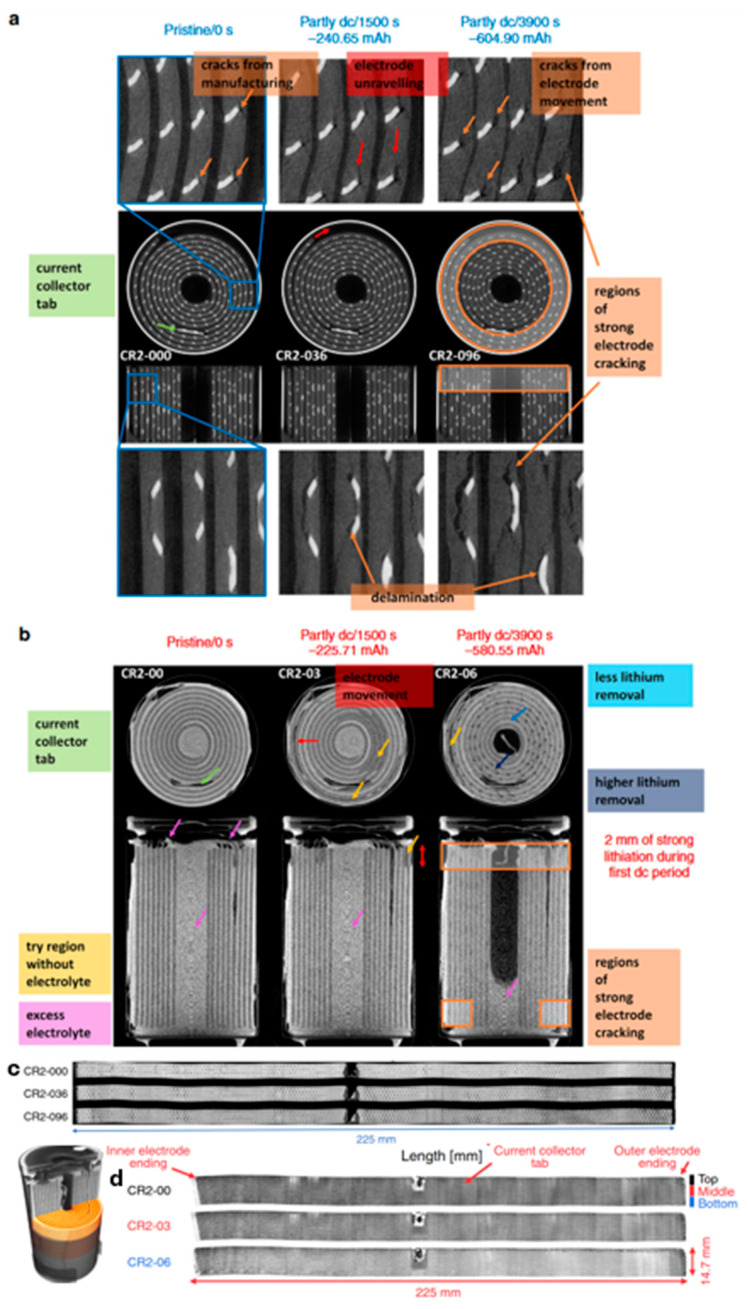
(a) X-ray and (b) neutron imaging of commercial Li/MnO_2_ cells that contain a wound electrode/separator/current collector assembly. The X-ray images are sensitive to the highly attenuating Ni current collectors, while the neutron technique is sensitive to the Li distributions and electrolyte. Cracks and delamination can be observed by X-rays in the cathode as the cell expands upon lithium insertion. Different colored arrows highlight regions of interest with features explained in the correspondingly colored text boxes. Virtual unfolding of the separator/electrode assemblies using (c) X-rays and (d) neutrons [88]. Reprinted with permission from [88]. Copyright 2020 Springer Nature.
